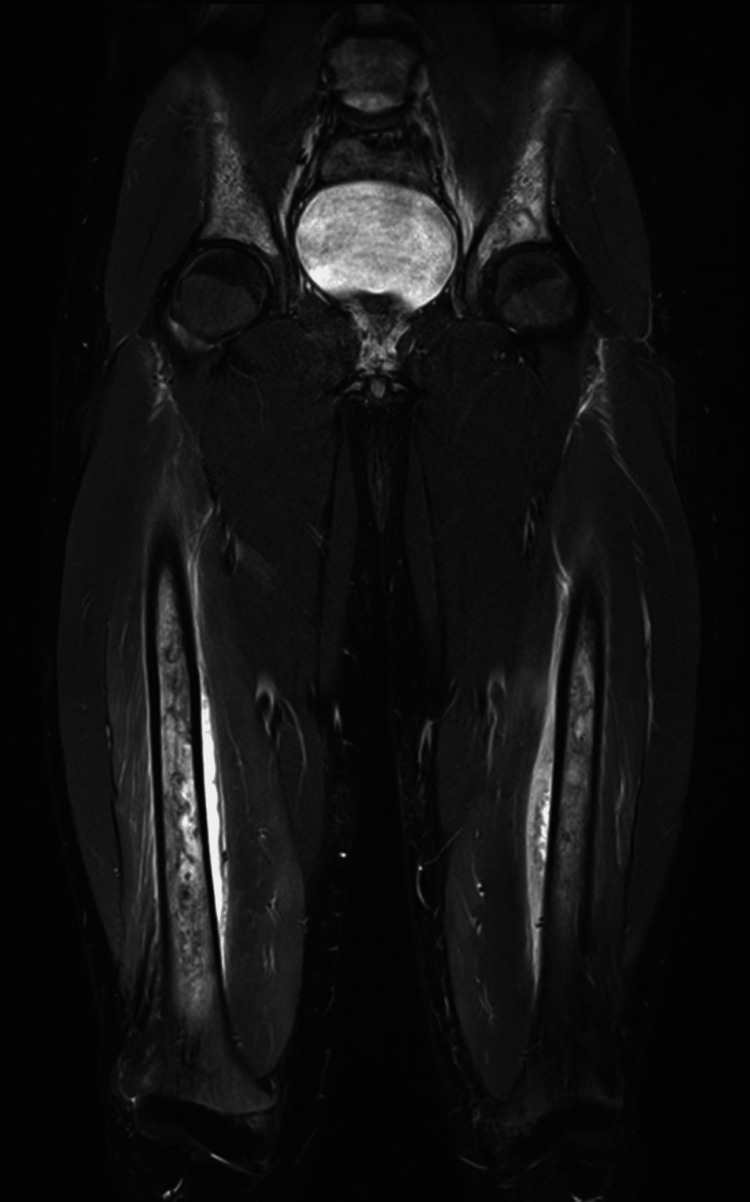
Day 10 coronal MRI scan A coronal T2 short inversion time inversion (STIR) image was taken on Day 10 after hospital admission. The image showed a significant reduction in muscle edema compared to the image taken on Day 6, however, the periosteal lifting and bone infarctions remained essentially unchanged.
